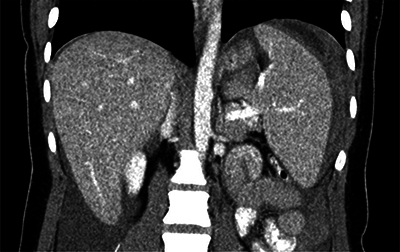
Contrast-enhanced abdominal CT shows a haemoperitoneum and splenomegaly. A hyperdense, perisplenic suggests an acute perisplenic haematoma.
